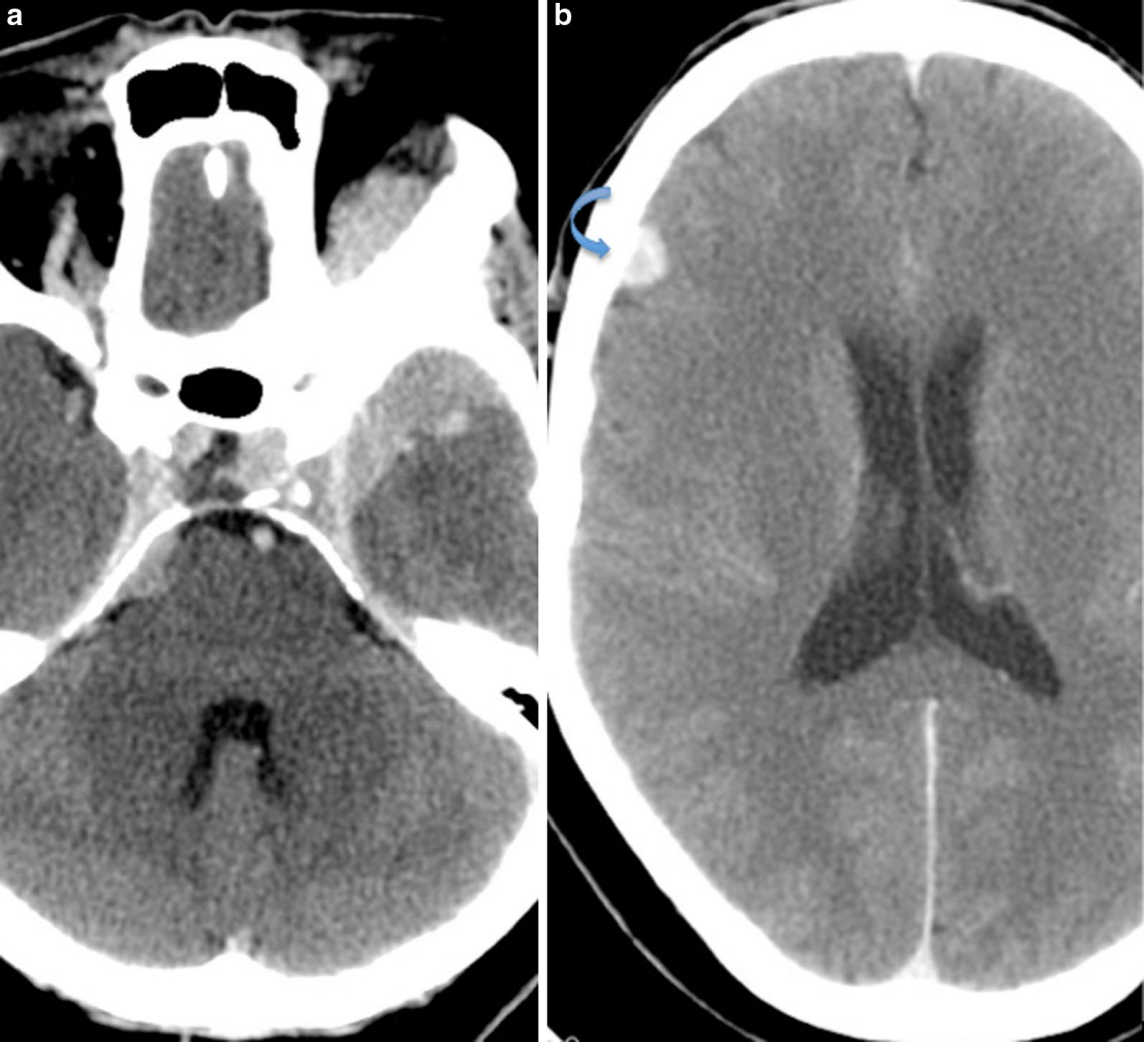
Axial contrast enhanced CT of the brain. a Large homogenously enhancing left sphenoid wing meningioma with associated hyperostosis and extension into the left anterior temporal fossa and left extraconal space. b Smaller well-defined enhancing extra-axial lesion in the right frontal lobe (*arrow*), consistent with a convexity meningioma
